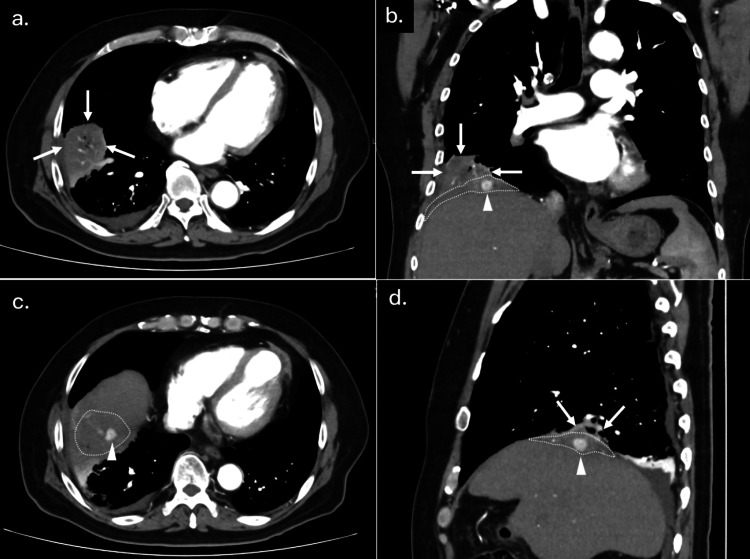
Following CT scan findings after antibiotic treatment (a-d) CT after antibiotic treatment showed the lung abscess (arrows), pneumonia, and subphrenic abscess (dotted areas) had improved. Arrowheads depict residual gallstone.
